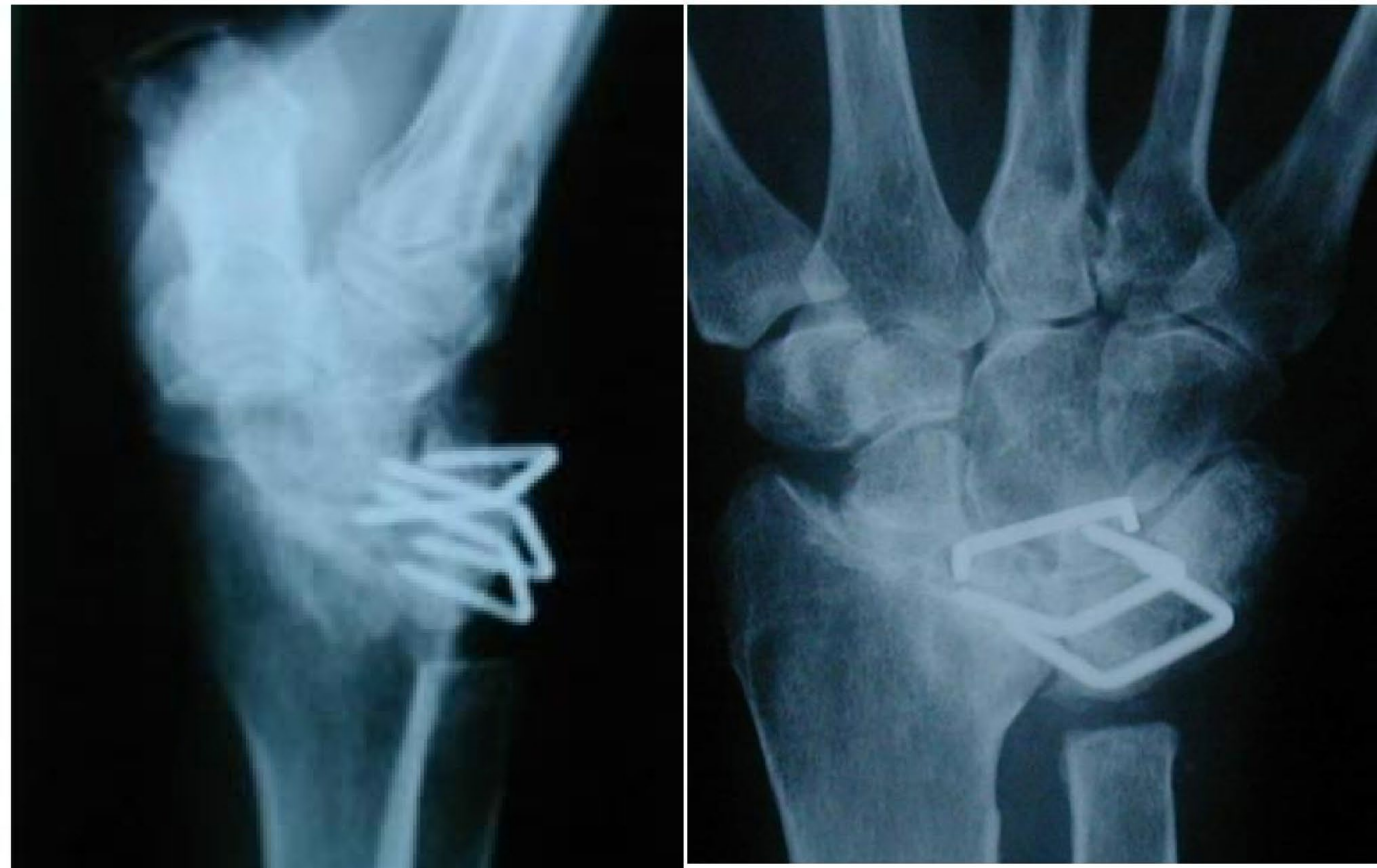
Case 4– Right preoperative X-ray in lateral and a.p. view
